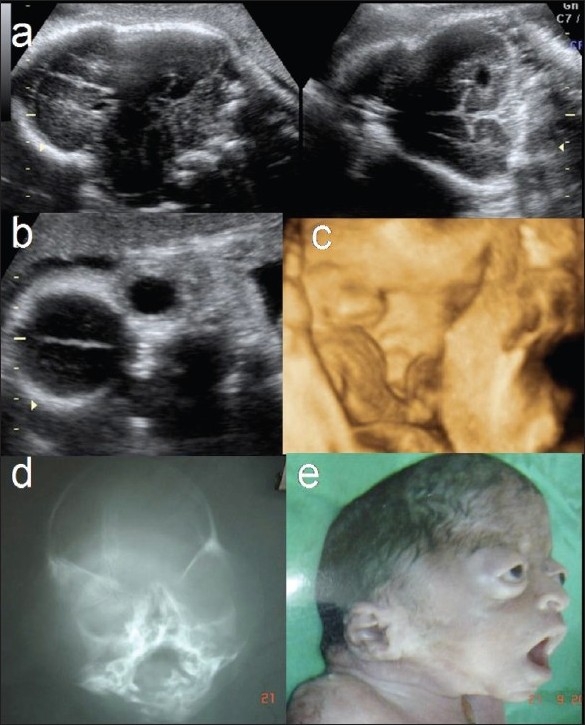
Crouzon syndrome. Axial and coronal sections of the fetal cranium (a) show a fused coronal suture and a clover-leaf calvarium. Coronal section of the face (B) shows prominent orbits. Surface rendering of fetal face (c) shows proptosis. Anteroposterior radiograph of the skull (d) and postabortal picture (e) of the abortus show typical featuresKlippel-Trenaunay-Weber syndrome: (sporadic) This syndrome typically features multiple soft-tissue hemangiomas in the subcutaneous plane. Other features include limb hypertrophy, and edema [Figure 8]. Differential diagnosis includes Proteus syndrome.
